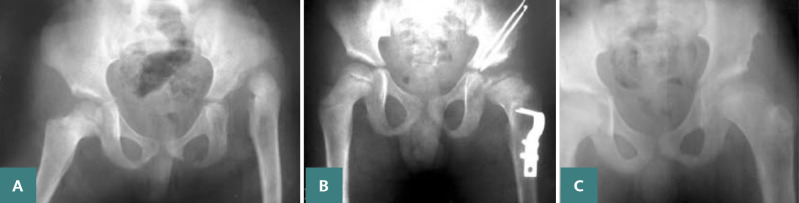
Post-operative X-ray of a patient who underwent open reduction, femoral shortening, and Salter osteotomy. A: Salter osteotomy, B: Post-operative X-ray showing a retroverted hip; Salter osteotomy with femoral graft. Removal of K-wire from bone transplant. C: X-ray outcome at 6 years classified as Severin class III.
